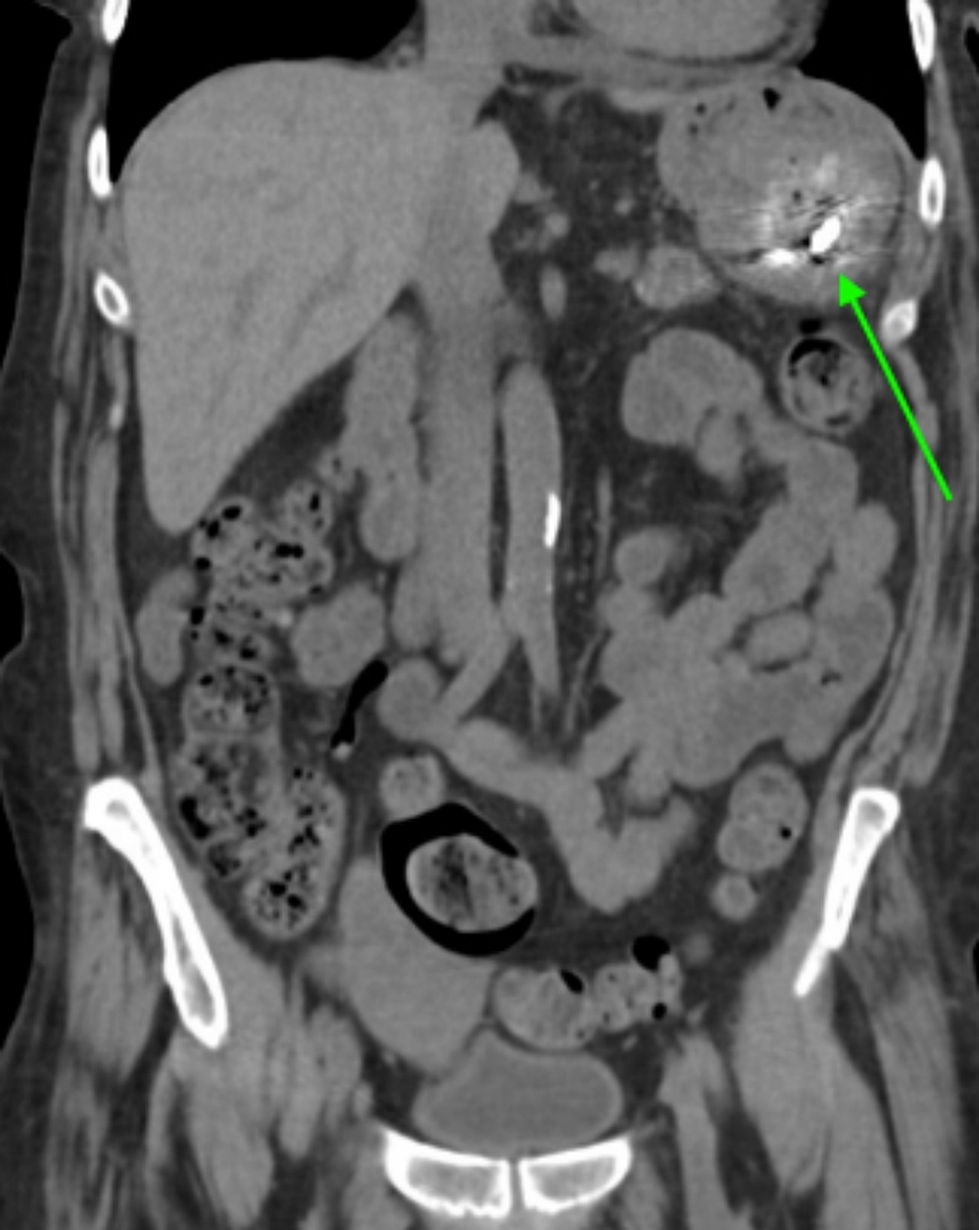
Coronal view CT of the abdomen and pelvis with intravenous contrast at three-month follow-up showing a drainage catheter (arrow) extending from the stomach to the collapsed pseudocyst within the left upper quadrant. There was no evidence of fluid re-accumulation. CT - computed tomography
